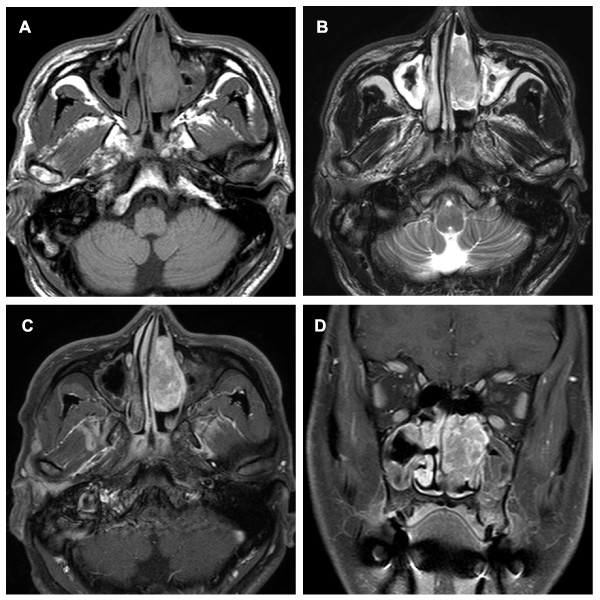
Magnetic resonance images before surgery. (A) T1-weighted PNS MRI showed a primary lesion of slightly lower signal intensity in the left nasal cavity. (B) The lesion was heterogeneously isointense on T2-weighted images. (C) Contrast-enhanced MRI showed heterogeneous enhancement of the lesion. (D) Coronal reconstruction of a T1-weighted MRI scan revealed an isolated primary lesion with no evidence of direct attachment to brain tissue.
